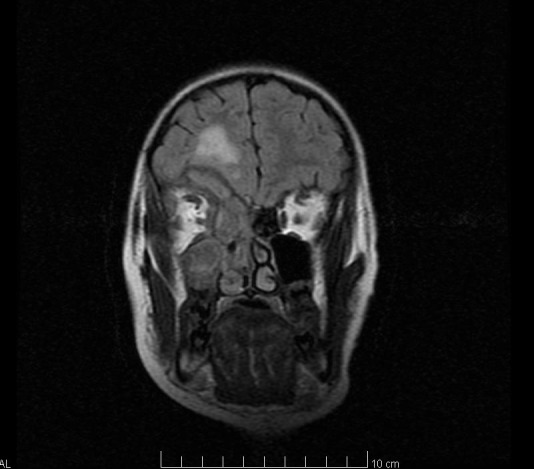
Preoperative Head MRI (blue arrow - orbital cellulitis, red arrow - sinusitis, yellow arrow - brain abscess)
